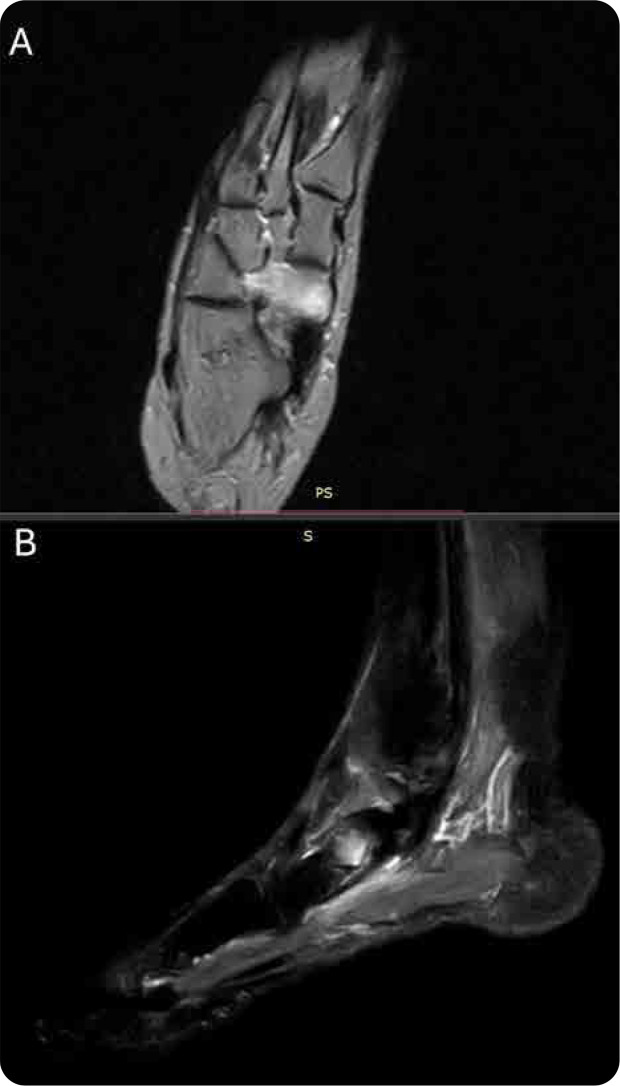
MRI images of the foot with T2-weighted (A) and STIR (B) sequences showing bone marrow oedema involving the entire navicular bone of the right foot.
